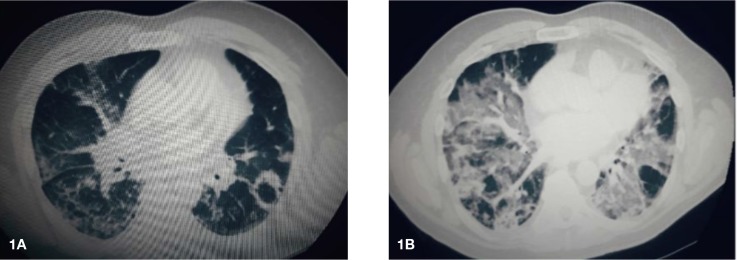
Chest Computed Tomography: A. Third day of hospitalization- bilateral circular reversed halo infiltrates, ground glass opacities mainly in right lower lobe. B. Day 39th since the first admission, CT showed significant progression of diffuse lung disease with many Ground glass opacities.
